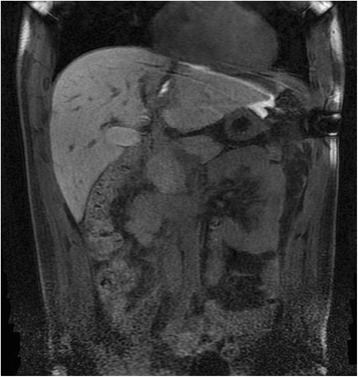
Magnetic resonance imaging (MRI) with hepatocyte-specific contrast agent showed a biliary leak originating from terminal branches of the left liver, with high signal of contrast collection under the left diaphragm
